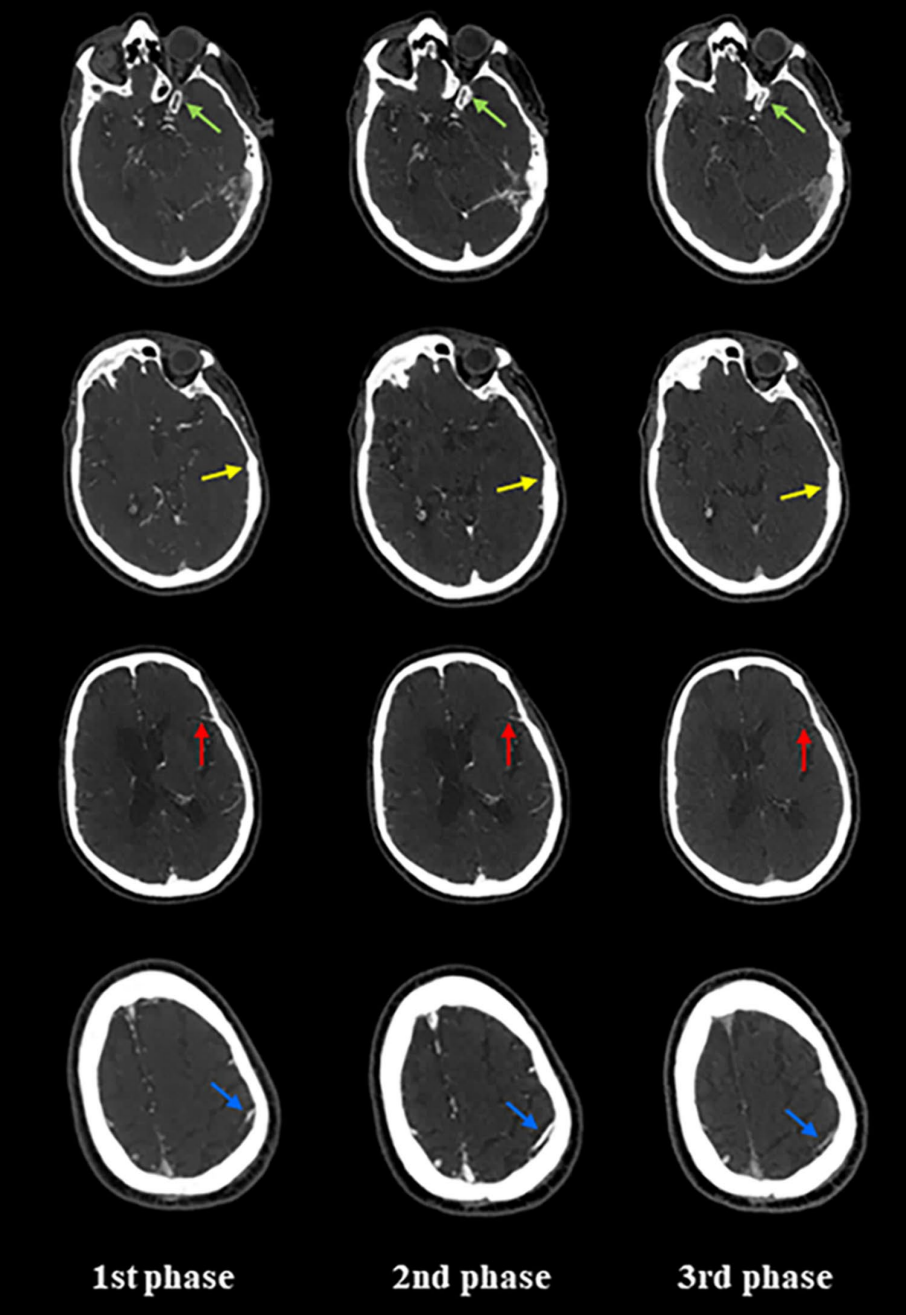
Opacification of sphenoparietal sinus (green arrows), superficial middle cerebral vein (yellow arrows) vein of Labbé (red arrows) and vein of Trolard (blue arrows) within the occluded territory in all three phases of multiphase CT angiography
